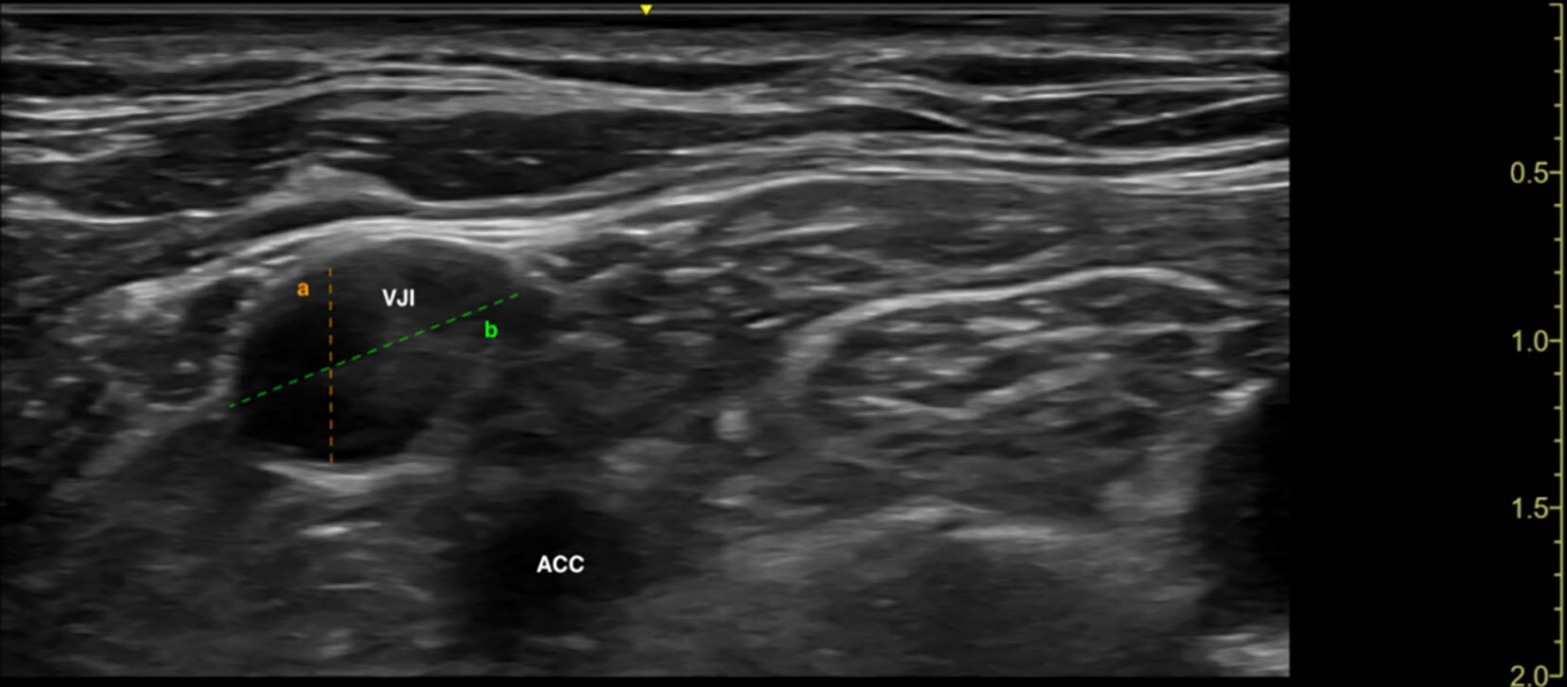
An ultrasound image of the vessel’s short axis (transverse plane). Line A is the diameter of the vessel for the conventional technique, and line B is the diameter for the novel technique. IJV vena jugularis interna, ACC arteria carotis communis
